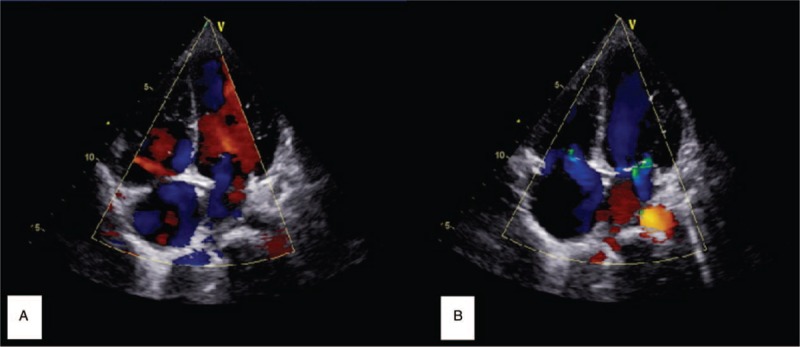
Echocardiography showed slight or moderate mitral regurgitation in the mitral valve (A) and in the tricuspid valve (B).
